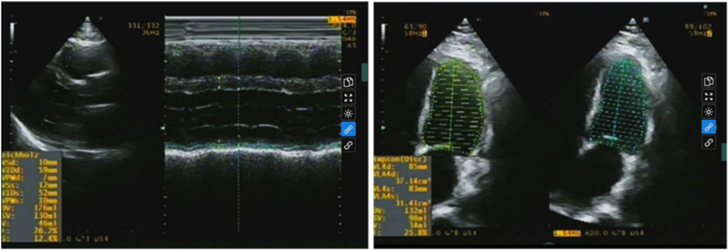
Transthoracic Echocardiography Apical 4-chamber view showing dilated left ventricle with global hypokinesis and reduced left ventricular ejection fraction (25% by Simpson biplane method).
